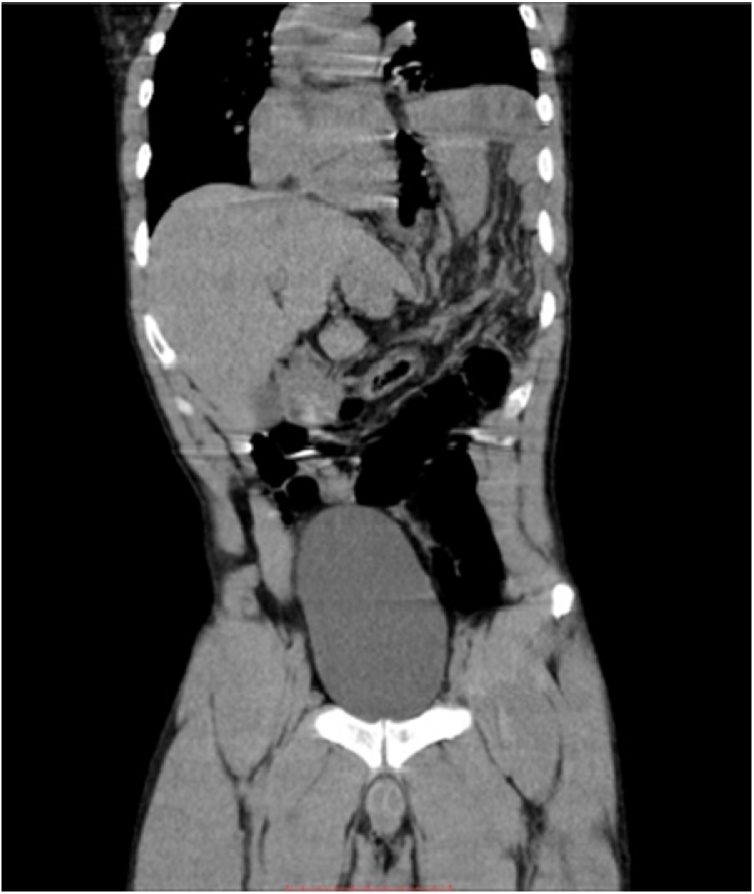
CT scan of the chest and abdomen showing voluminous left diaphragmatic hernia.
